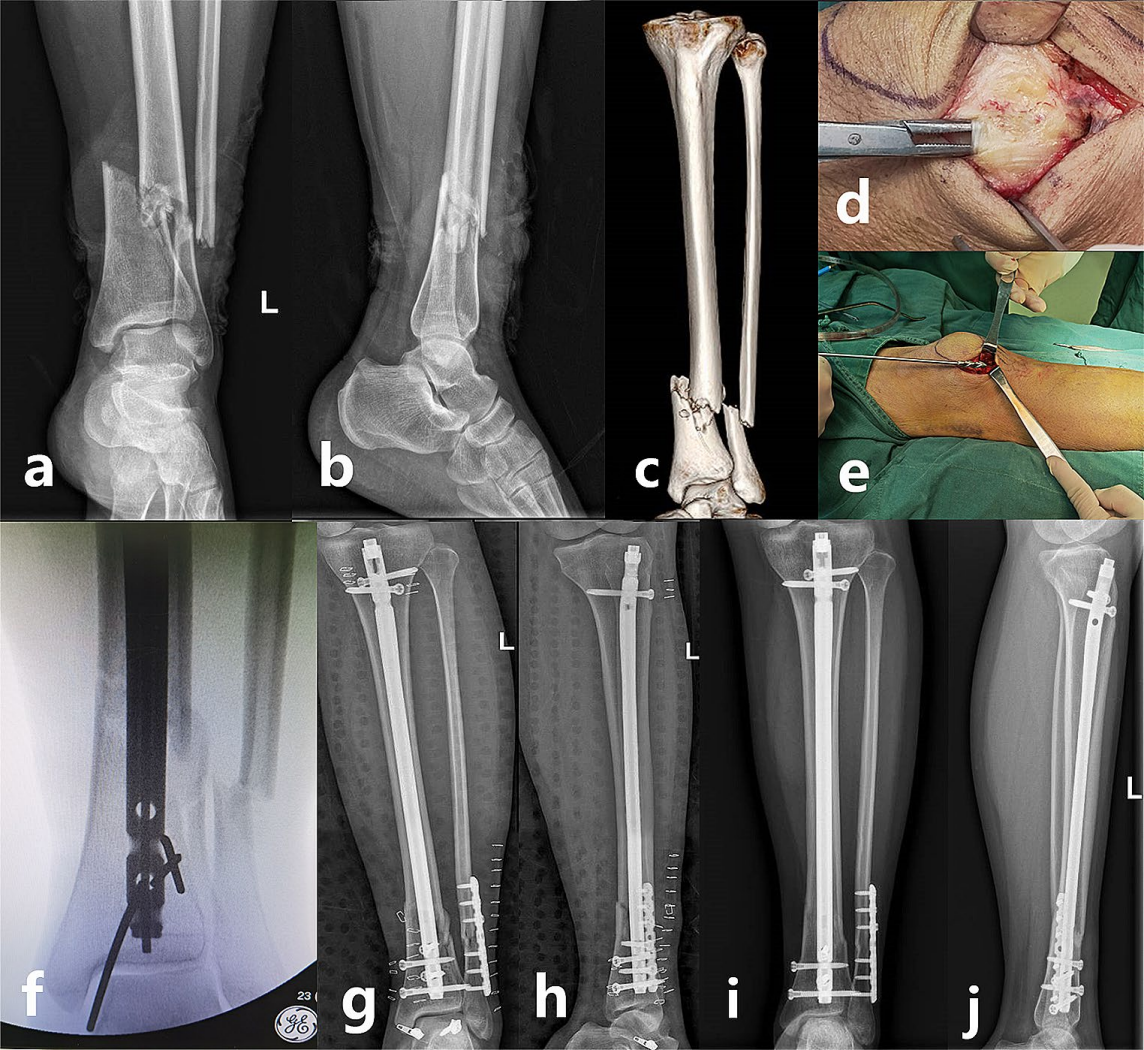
Preoperative anteroposterior (a) and lateral (b) plain radiographs and the 3-dimensional reconstruction CT scan (c) were taken for a 38-year-old man who sustained a distal tibial fracture and a concomitant fibular fracture in the left side. He experienced the semi-extended tibial nailing through the lateral parapatellar extraarticular approach. The intraoperative photograph shows that the retinaculum was separated from the underling joint capsule (d). A rigid handled reamers was used to drill the proximal metaphysis so as to keep the right alignment of reaming (e). K-wires were used as blocking screws for fracture reduction (f). Satisfactory reduction was confirmed on the postoperative plain radiographs (g, h) two days after surgery. Bony union was noted on the plain radiographs (i, j) at the 5-month follow-up
